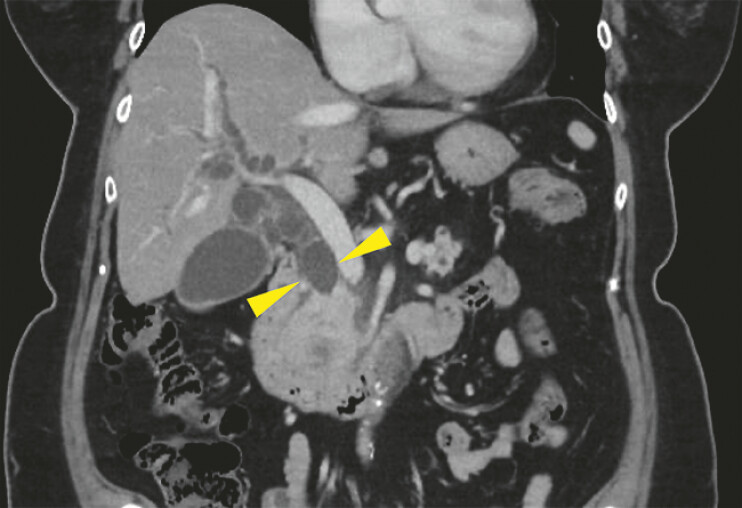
Pre-procedural computed tomography scan demonstrating a dilated common bile duct (yellow arrowhead).
